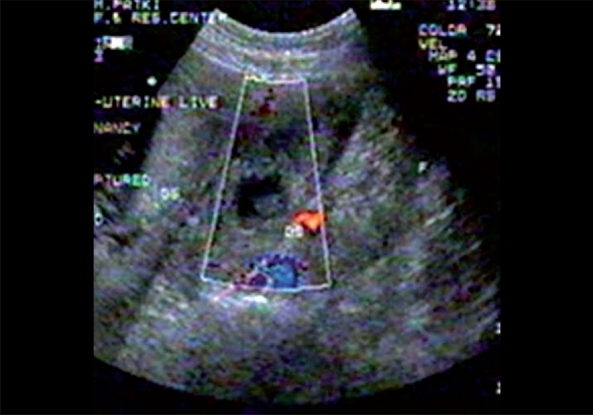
TVS color Doppler showing adnexal mass with color flow in the trophoblastic tissue
